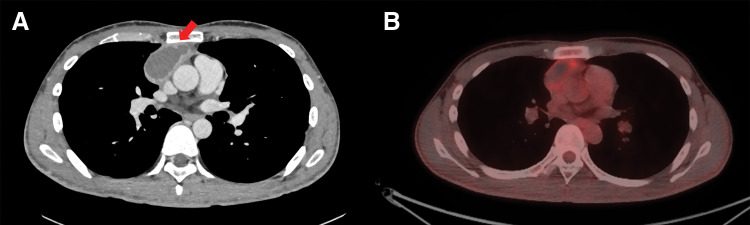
Case 2. Finding of chest CT. (A) Chest CT showed an 88 × 60 × 29 mm cystic tumor in the anterior mediastinum with contrast enhancement in the walls (arrow). Pleural effusion was not observed. (B) ^18^F-fluorodeoxyglucose positron emission tomography (FDG-PET) computed tomography shows FDG uptake with a maximum standardized uptake value of 3.2.
